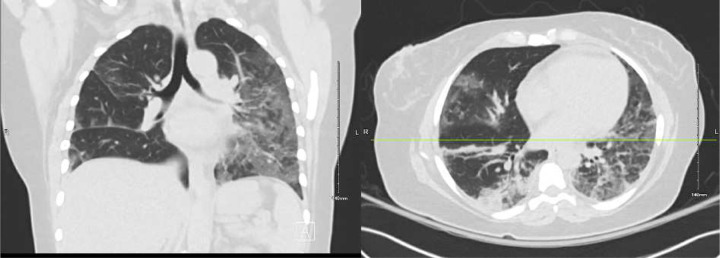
Chest computed tomography without contrast (admission 1, day 2), showing multifocal, mixed airspace and ground glass opacities extending towards the periphery of the lungs.
